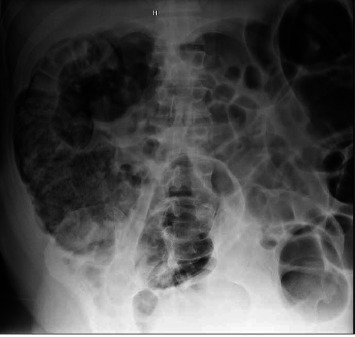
Plain abdomen x-ray showing proximal colonic dilatation.
